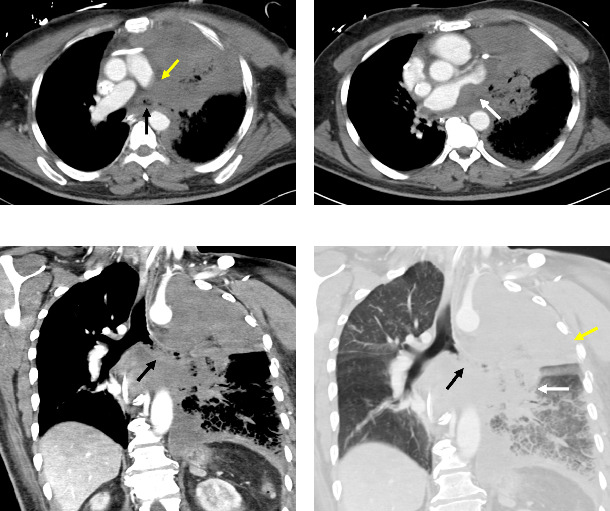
A 59-year-old male with AML undergoing chemotherapy developed fever, cough, pleuritic chest pain, and eventual respiratory failure requiring intubation due to pulmonary mucormycosis that evolved into disseminated disease. Axial views of CT chest images with IV contrast in soft tissue window 11 days later (a and b) show persistent consolidation of the left lung with left main stem bronchus occlusion (black arrow) and complete thrombosis of the left main pulmonary artery (yellow arrow) with absent pulmonary arterial flow to the left lung. There is soft tissue infiltration in the mediastinum with mass effect on the left atrium (white arrow). Coronal views in (c) soft tissue window and (d) lung window show occlusion of the left main stem bronchus with heterogeneous intraluminal material (black arrow) with complete left upper lobe consolidation (yellow arrow) and left perihilar consolidation (white arrow) with diffuse ground-grass opacity in the left lower lobe.
